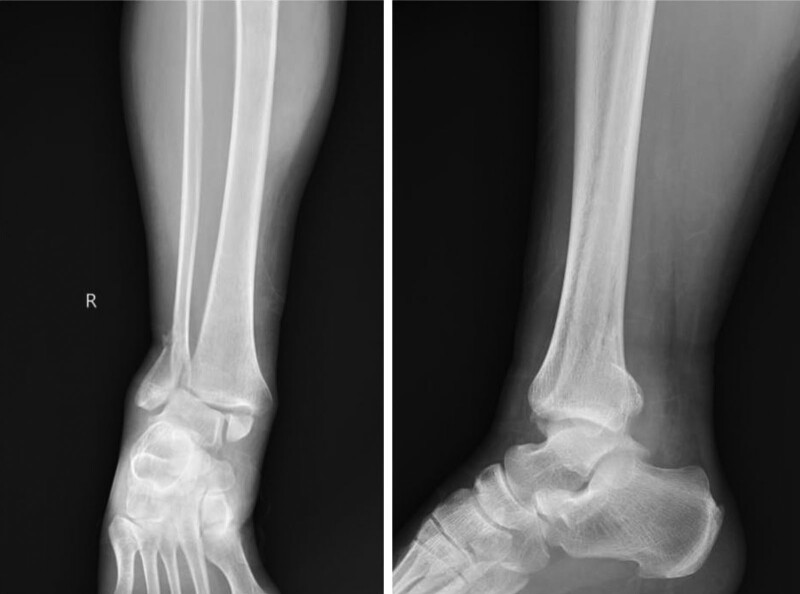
X-ray imaging after injury. It displayed trimalleolar fracture accompanying ankle dislocation.
